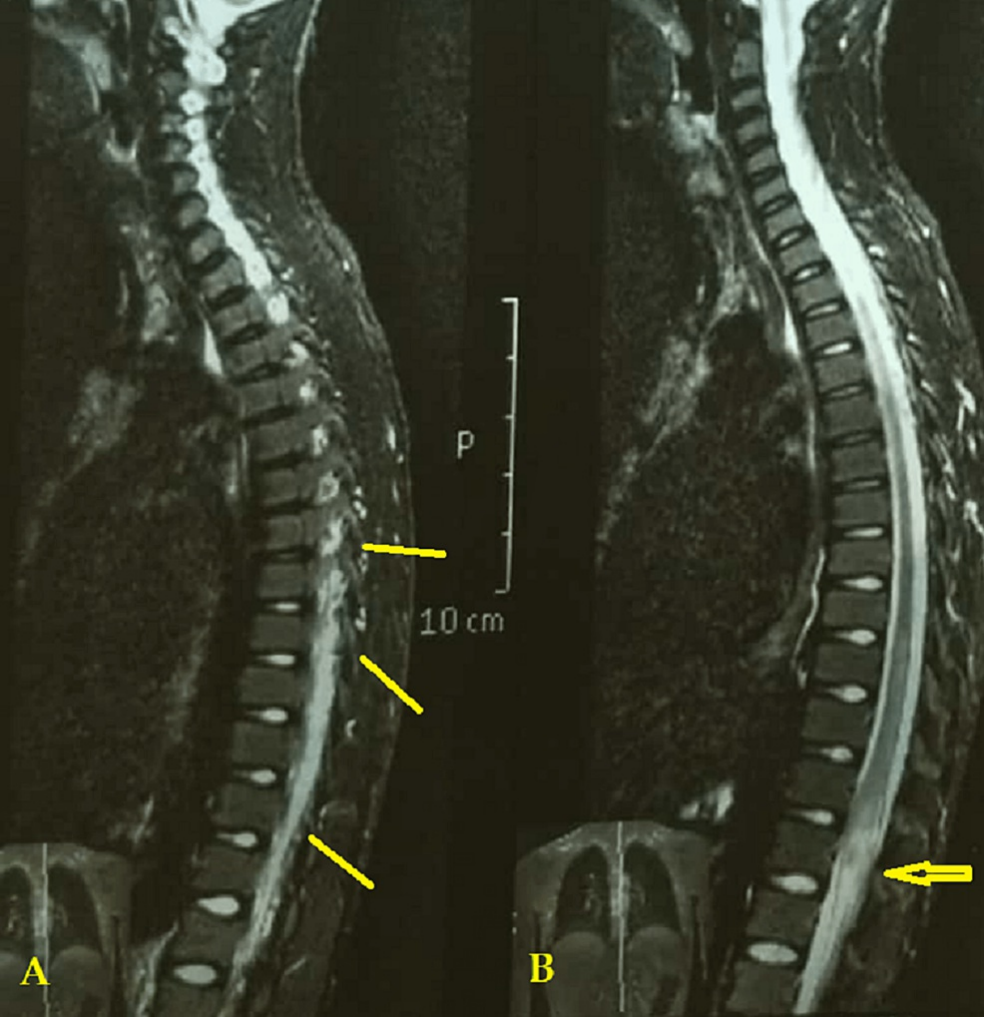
Detailed MRI observations Magnetic resonance imaging showing: A; an impregnation of contrast of the cauda equina with heterogeneous pattern (arrow). B; a dilated conus medullaris (arrow).
